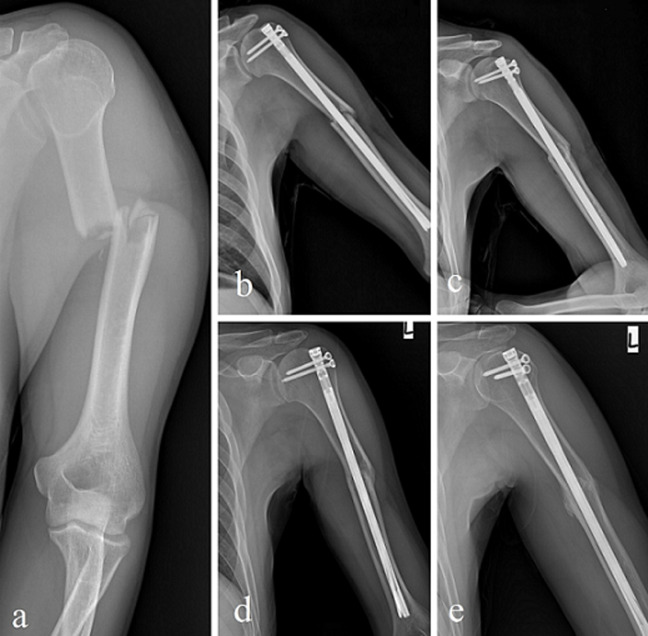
IMN fixation, X-ray images of a 43-year-old man with a left humeral shaft fracture as a result of a traffic accident: (a) initial anteroposterior (AP) plane X-ray image; (b and c) early postoperative X-ray images; (d and e) X-ray images at 6^th^-month follow-up after surgery
